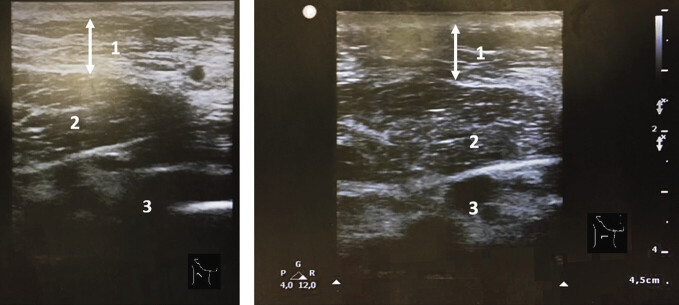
Sonografie der Kopf- und Halsweichteile rechts zervikal Level III. Diffuse Weichteilschwellung (1), Musculus sternocleidomastoideus (2), Halsgefäßnervenscheide mit Vena jugularis interna (3).
